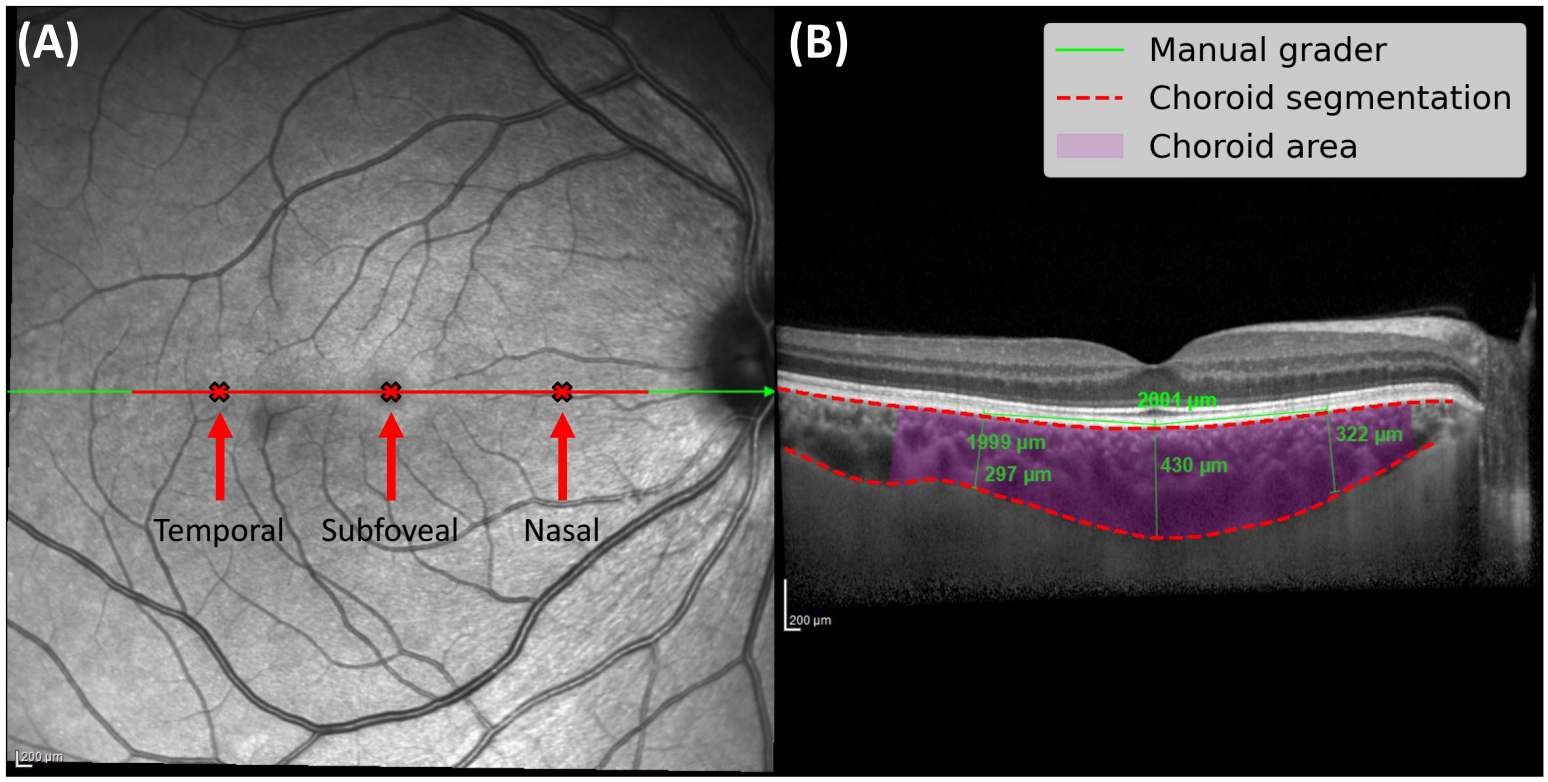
EDI-OCT scan of a donor's right eye at baseline. (A) En face view of the fundus with the location of the B-scan in *green* and markers for measuring CT and CA in *red*. (B) B-scan showing chorioretinal structures with manual and automated assessment shown.
